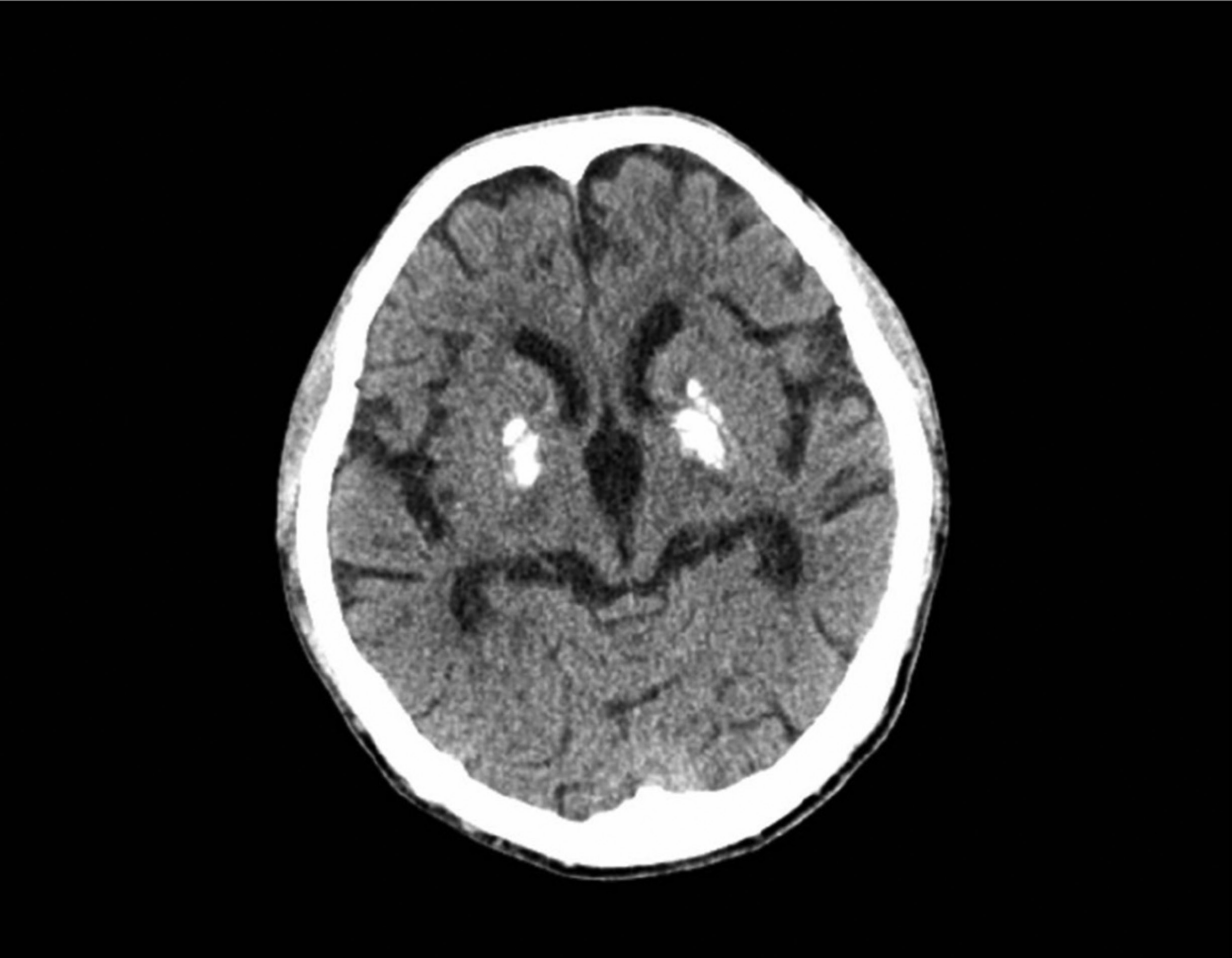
Head computed tomography (CT) scan revealing calcification in bilateral basal ganglia.
